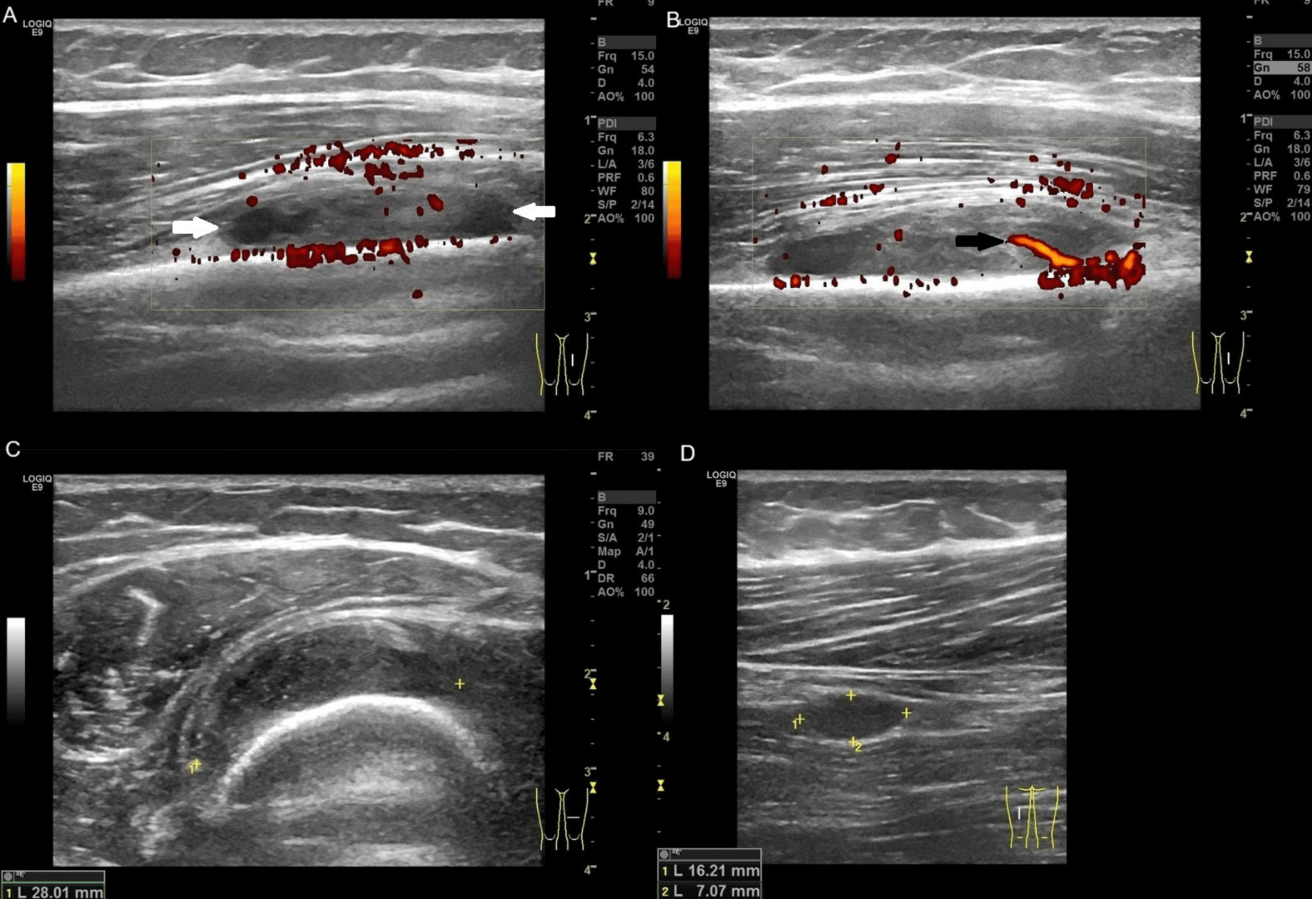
Ultrasound images of the left thigh. Longitudinal color Doppler (A-B) ultrasound images of the thigh show a fusiform, heterogeneous soft tissue mass with peripheral vascularity. A feeding vessel is seen (B, black arrow). Note that echogenicity is markedly lower at the poles of the mass (A, white arrows). Transverse ultrasound images (C-D) show an intramuscular mass of mixed, mostly low echogenicity, in the thigh.
